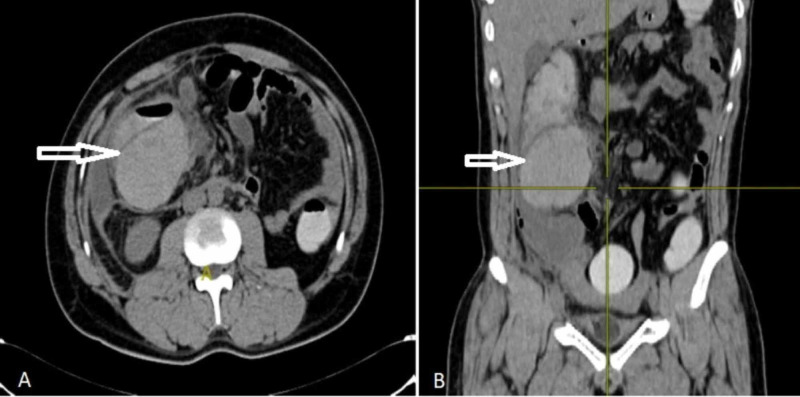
Contrast-enhanced computed tomography (CECT) abdomen with oral and rectal contrast showing intramural ascending colon hematoma. (A) Coronal view and (B) sagittal view.
